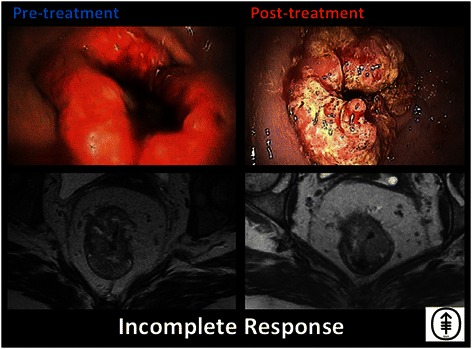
Incomplete response. Endoscopic and T2-weighted MRI images both pre- and post-treatment are shown for a patient who experienced no significant response to induction chemotherapy followed by CRT. This is a 45-year-old woman who underwent 8 cycles of induction FOLFOX followed by CRT with minimal or no response. The patient was therefore referred for TME
